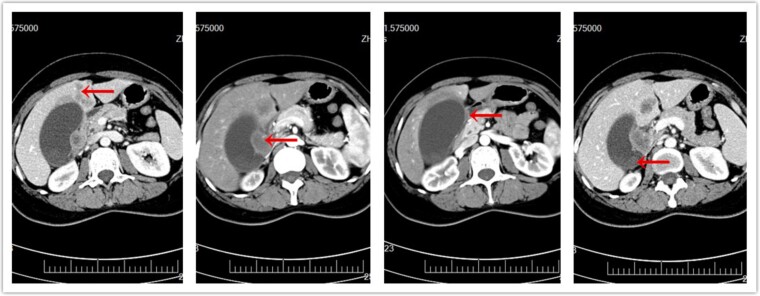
CT of abdomen prior to surgery. (a) Metastatic liver masses. (b) Enlarged lymph nodes. (c) Invasion of duodenum. (d) Original gallbladder tumor.
